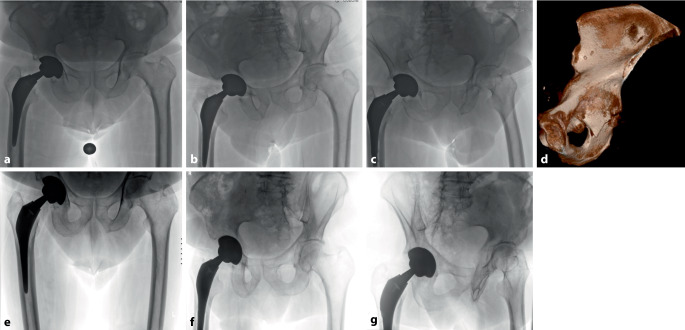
Aufnahmen einer 74-jährigen Patientin. a–d Rechtsseitige periprothetische Hinterpfeiler-Acetabulum-Fraktur und stabile Prothese nach Sturz aus Körperhöhe; e-g Verlaufskontrolle nach 6 Monaten konservativer Therapie mit vollständig konsolidierter Fraktur und stabilem Implantat (a, e a‑p.; b, f Ala, rechts; c, g Obturator, rechts; d CT-basierte 3D-Rekonstruktion mit Darstellung der Fraktur, Blick von intrapelvin)
